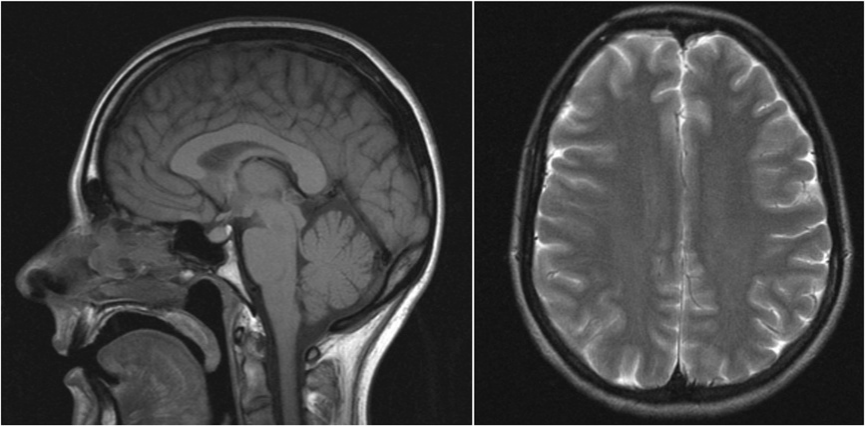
Intracranial magnetic resonance imaging (axial: T2-weighted and saggital: T1-weighted sections) performed at one month after discharge shows normal intracranial appearances. The bilateral extra-axial collections and cerebellar descent have resolved.
